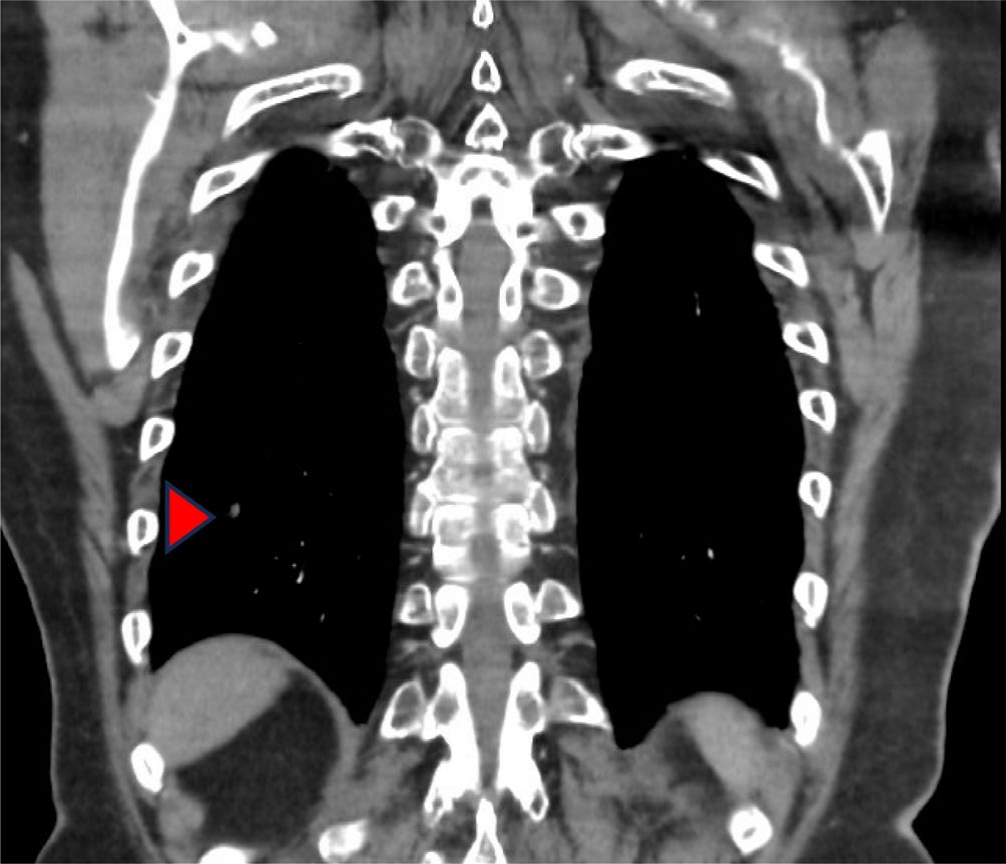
FDG PET‐CT scan showing significant resolution of 2× metastatic lesions along the oblique fissure (see arrow) of the right lobe seen in Figure 2 following treatment with pembrolizumab and pazopanib.
